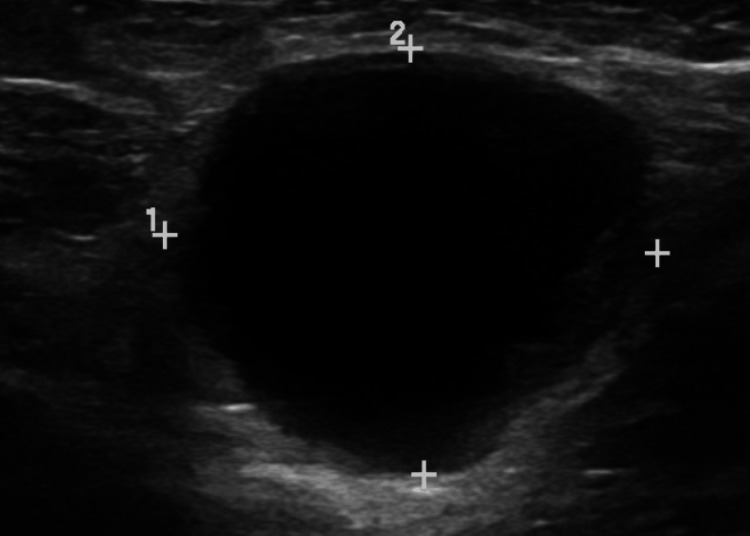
Ultrasound of left axillary mass performed on LOGIQ S8. Measurement 1=2.62 cm, measurement 2=2.27 cm.
